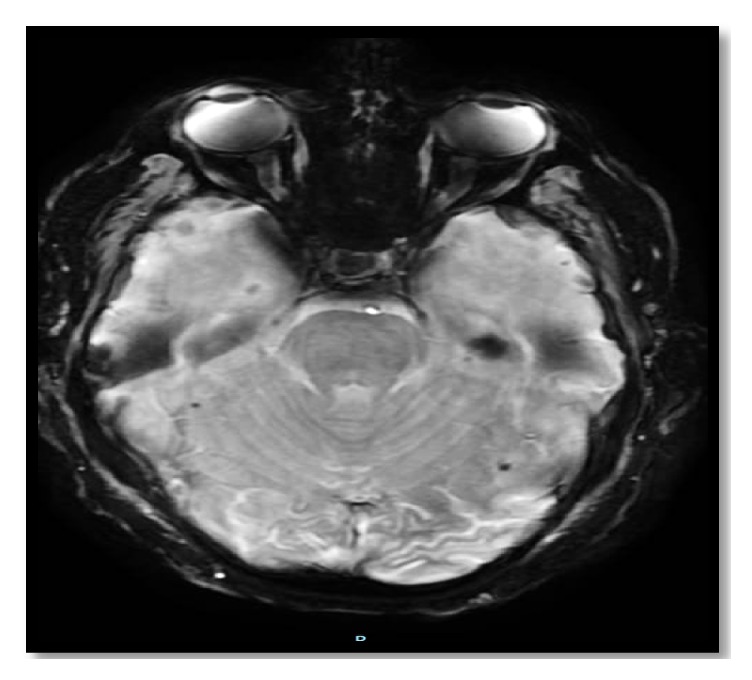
Periictal brain MRI SWAN image: showing gyriform hyperintense signal abnormality over bilateral occipital cortex. Abbreviations: MRI, magnetic resonance imaging; SWAN, susceptibility weighted angiography.
